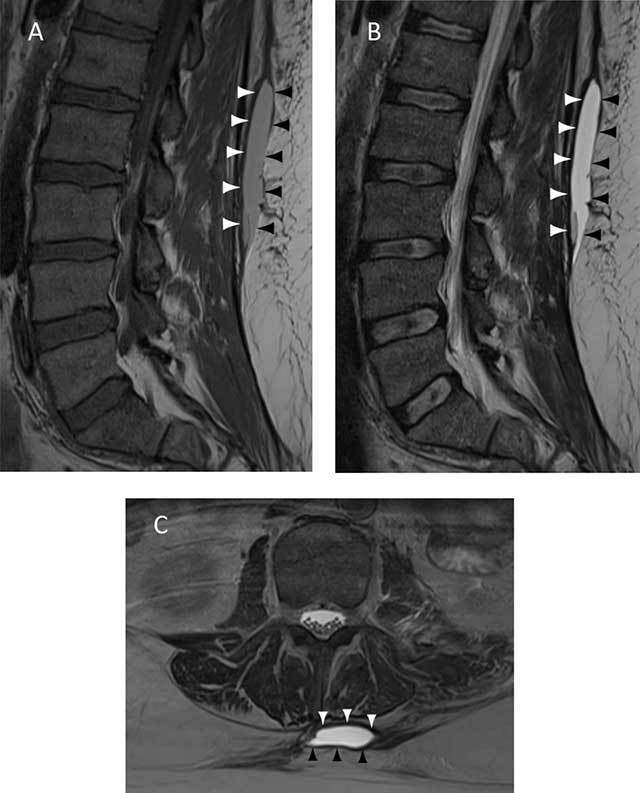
A 51-year-old man with low back swelling status post trauma. Type II lesion: subacute hematoma of the lumbar region. Sagittal T1 (A), sagittal T2 (B) and axial T2 (C) MR images of the lumbar region demonstrate a collection between the thoracolumbar fascia (white arrowheads) and the overlying subcutaneous fat and superficial fascia (black arrowheads). This subacute hematoma has a homogeneously high SI on T1-WI relative to muscle, consistent with methemoglobin.
